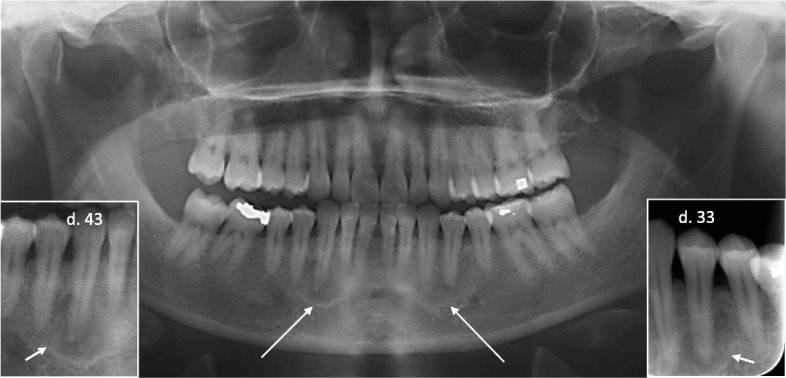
Orthopantomogram and two intraoral radiographs (insets) of a 35-year-old woman with NF1 shows periradicular radiolucencies with intralesional calcification around vital teeth number 33 and 43 (arrows) suggesting the mature stage of periapical cemental dysplasia
